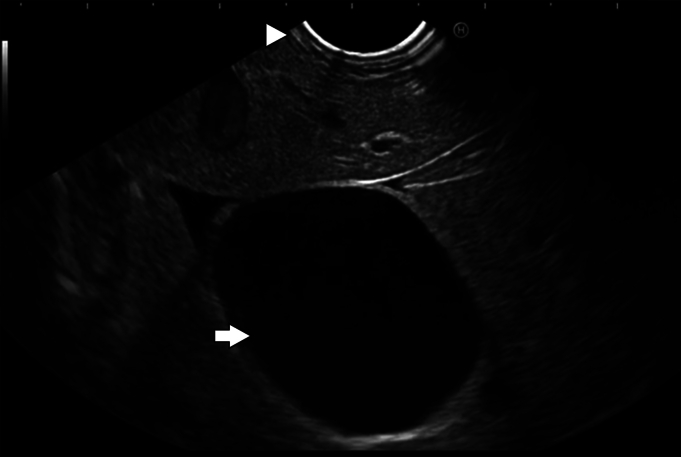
Gallbladder (*arrow*) visualized endosonographically, notably in a location far from the gastric wall (*arrowhead*).
